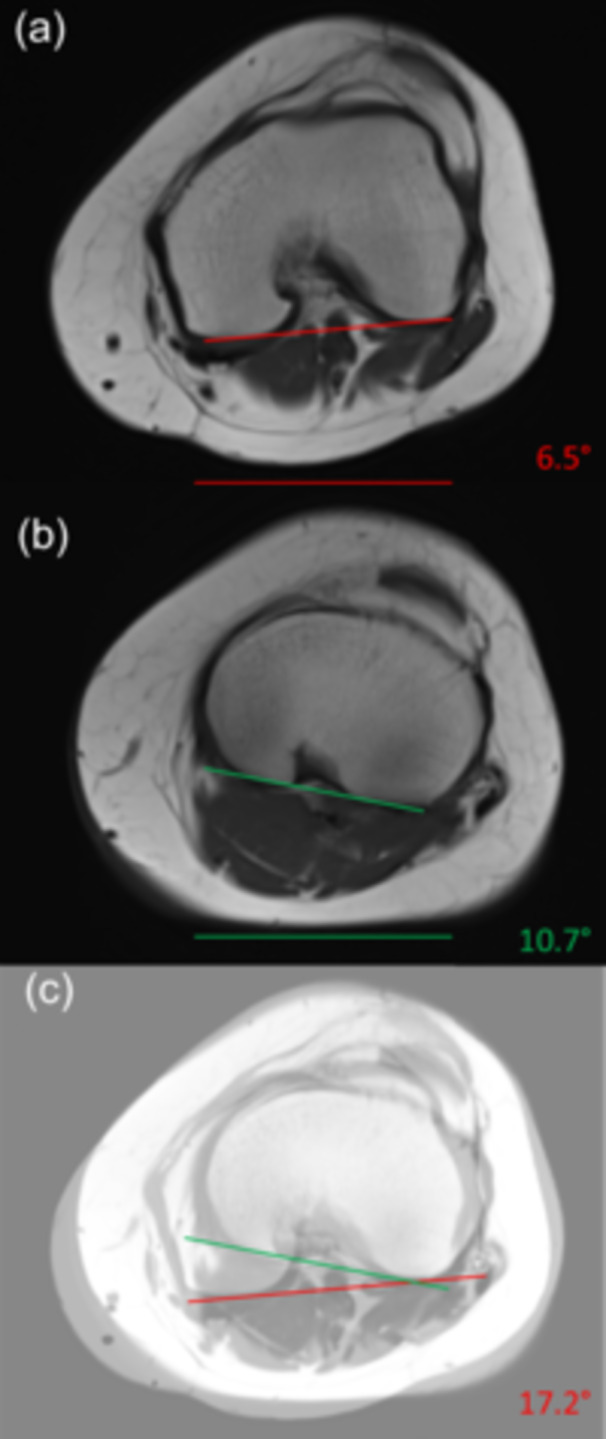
Demonstration of the measurement of the tibiofemoral rotation according to Eckhoff et al. [12]. Tibiofemoral rotation was assessed using axial T2‐weighted MR images. (a) At the distal femur, a line (red) was drawn connecting the posterior aspects of the femoral condyles. (b) At the proximal tibia, a line (green) was drawn along the posterior cortical margin of the tibial plateau. (c) The angle between the femoral and tibial lines results from overlaying a and b and represents the tibiofemoral rotation, calculated as the angle between the posterior femoral condylar axis and the posterior tibial axis.
